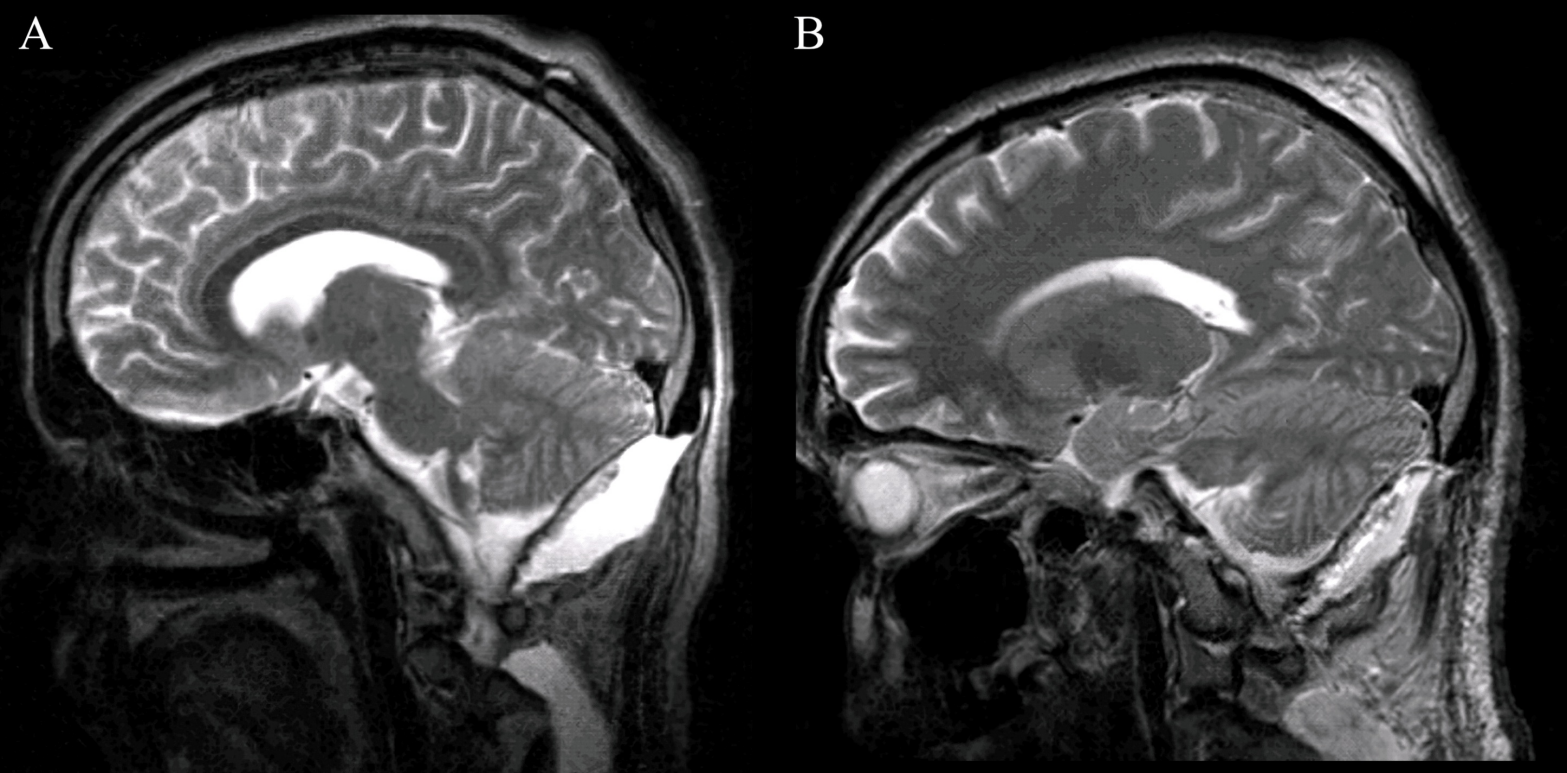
(A, B) Sagittal brain MRI obtained nine months postoperatively demonstrating postoperative changes in the posterior fossa without evidence of residual or recurrent lesion.
